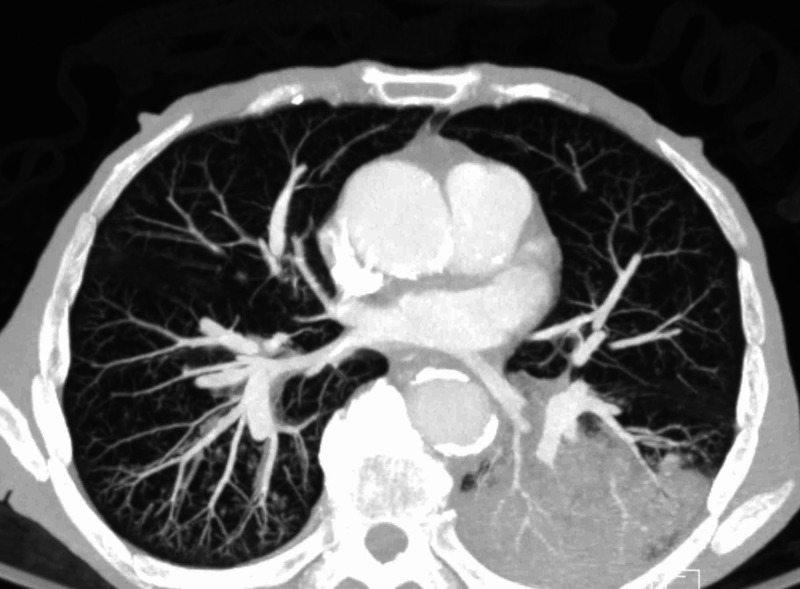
CT angiogram of the chest shows significant left lower lobe infiltrate CT: computed tomography
